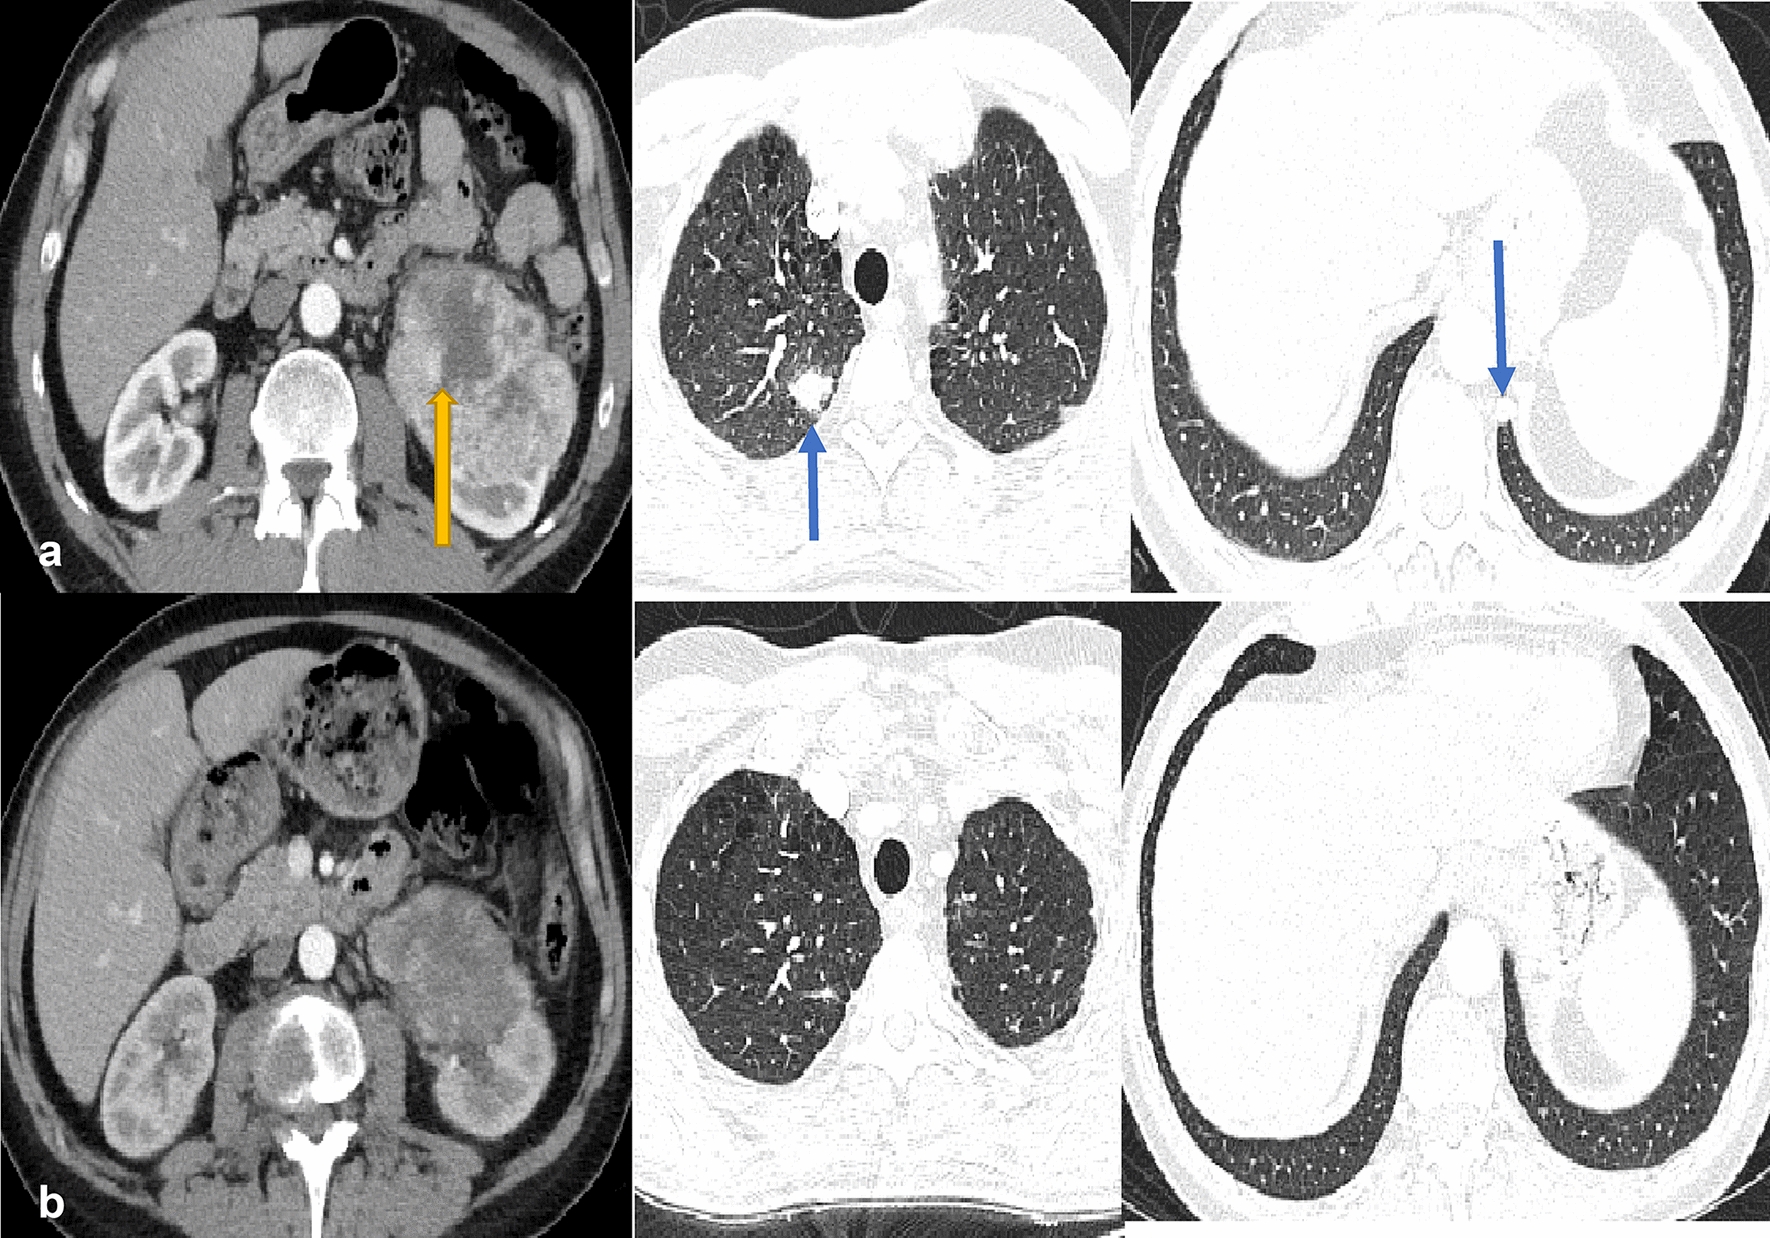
A, B Axial contrast-enhanced CT images of the abdomen (soft tissue kernel) and chest (lung kernel) obtained at baseline (A, May 2019) and follow-up after treatment (B, September 2019). Large heterogeneous enhancing left renal exophytic mass representing the known primary renal cell carcinoma (yellow arrow), decreasing in size on the follow-up examination (B), with resolution of the lung metastases in the right upper and left lower lobes (blue arrows). Imaging findings were consistent with favorable response to therapy
